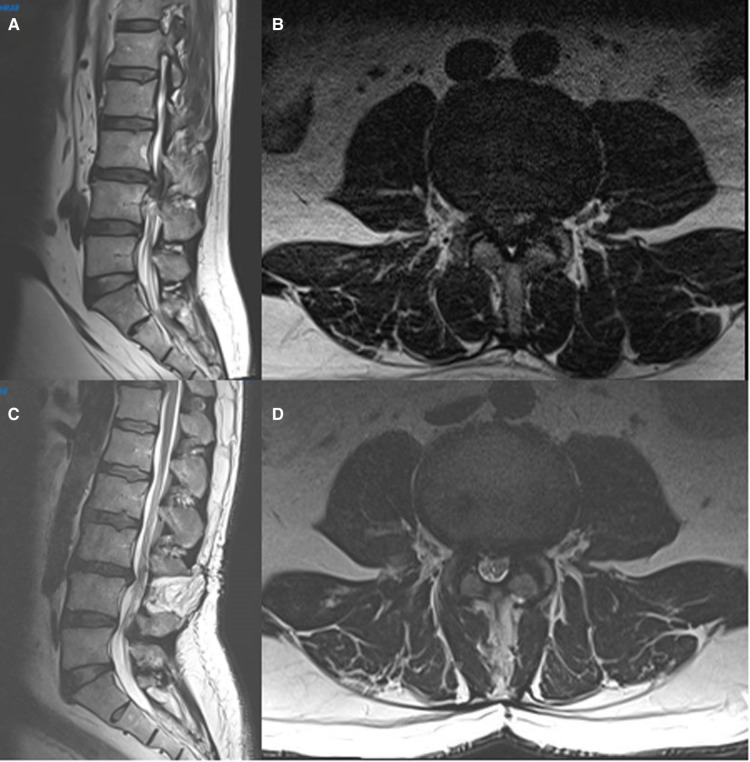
Preoperative (A,B) and postoperative (C,D) sagittal and axial MRI images of a typical patient diagnosed with an CES due to a giant L3–4 disc herniation and operated by open laminectomy and discectomy.
